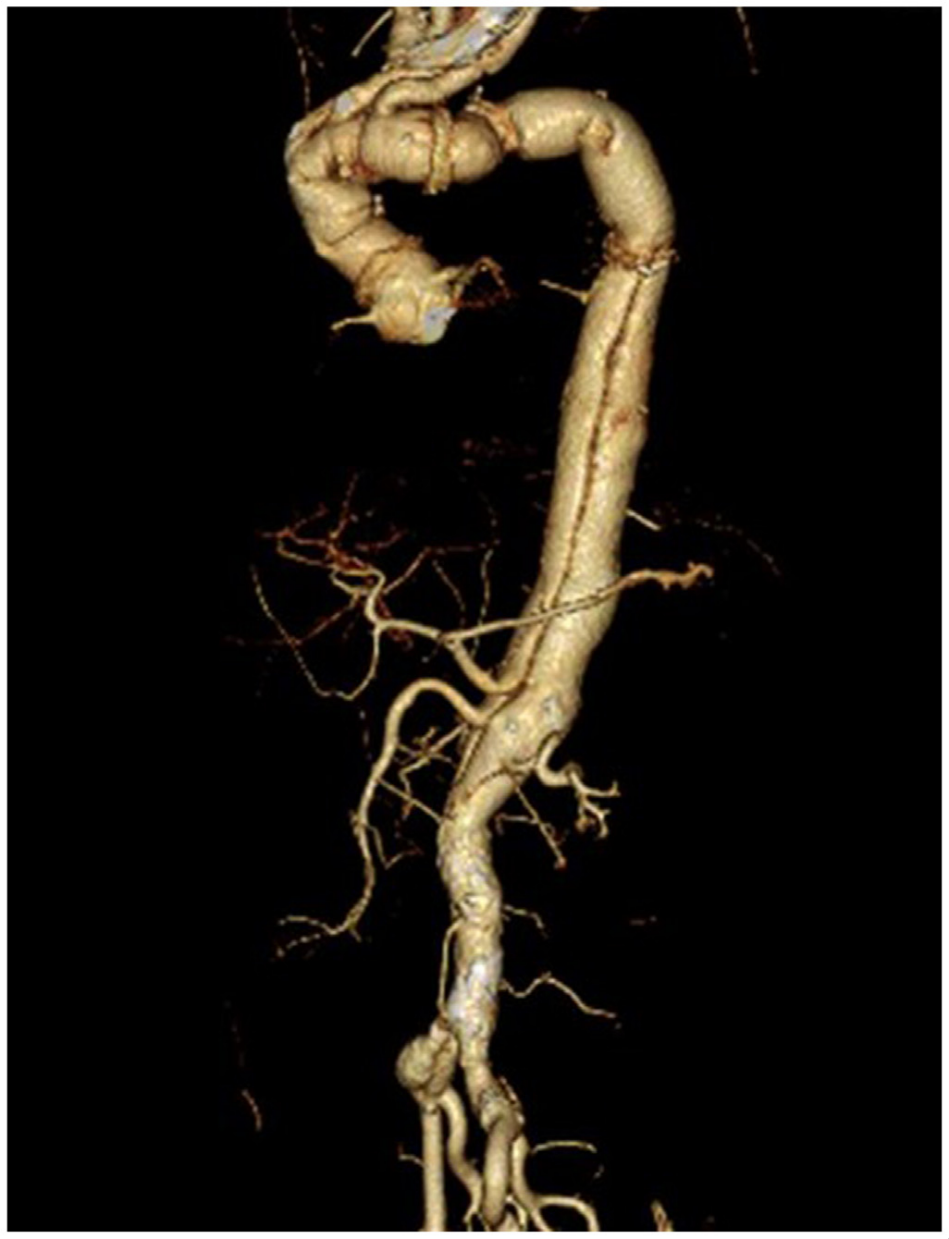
Postoperative contrast computed tomography (*CT*) scan. A three-dimensional (3D) reconstruction of a preoperative computed tomographic image at the 1-year follow-up showing good perfusion of both lumens. No aneurysmal dilatation in the aorta peripheral to the double-barreled anastomosis is observed.
